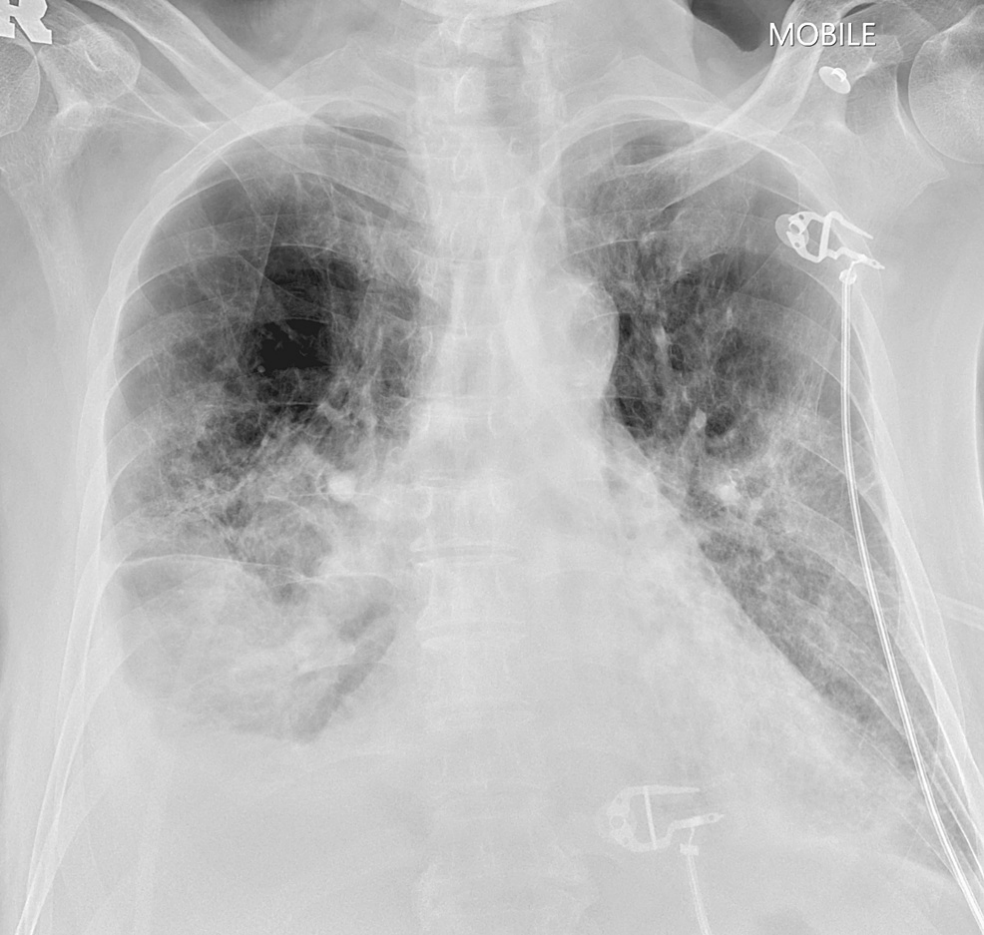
Chest X-ray showing bilateral lower zone consolidations with right pleural effusion
